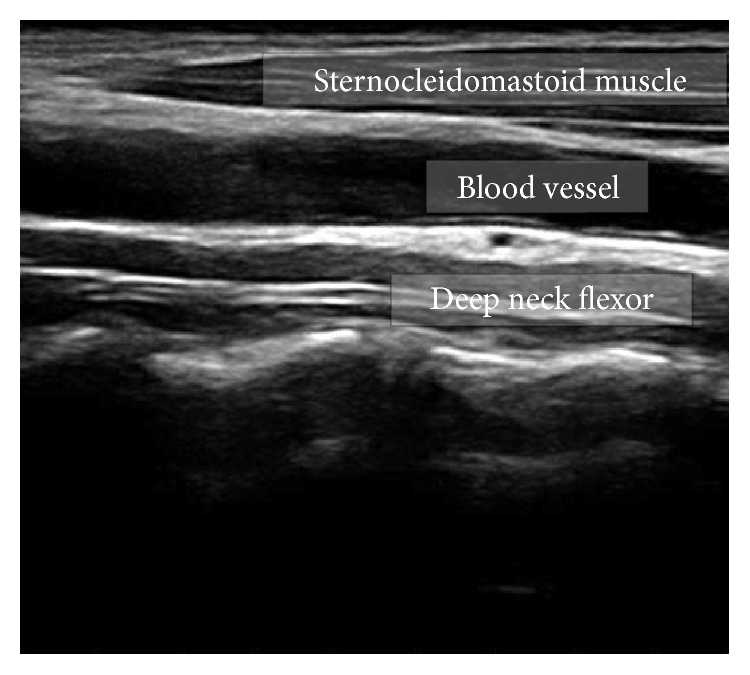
ROI of ultrasound image.
